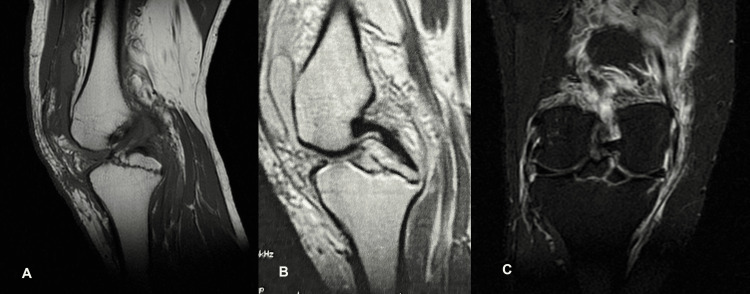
MRI of the patient's injured knee displaying the ACL-PCL avulsion fracture. (A-B) MRI sagittal view. (C) MRI coronal view. MRI: magnetic resonance imaging; ACL: anterior cruciate ligament; PCL: posterior cruciate ligament
